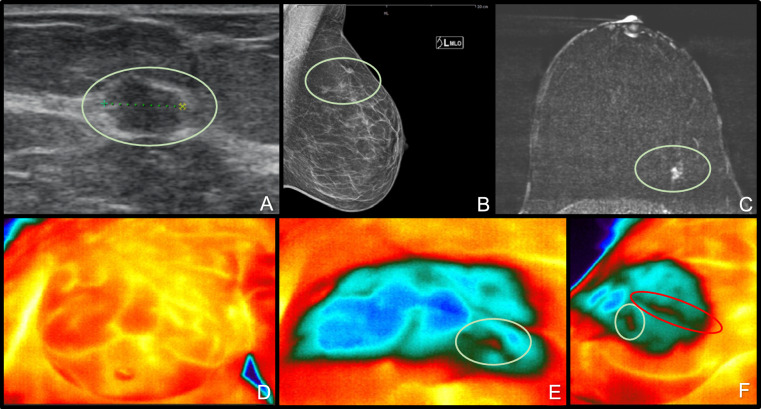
Top row left to right: Sonography (A), Mammography mediolateral-oblique view (B); Breast Computer Tomography (C); bottom row left to right: Heat flow imaging: native view (D), frontal view pad €, 45°angel view (F); the green circle show the carcinoma; the red circle a blood vessel
